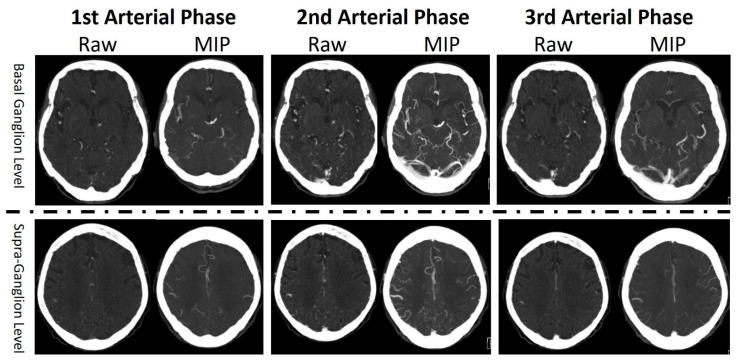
Demonstration of 12 images selected from each multiphase CTA study.
